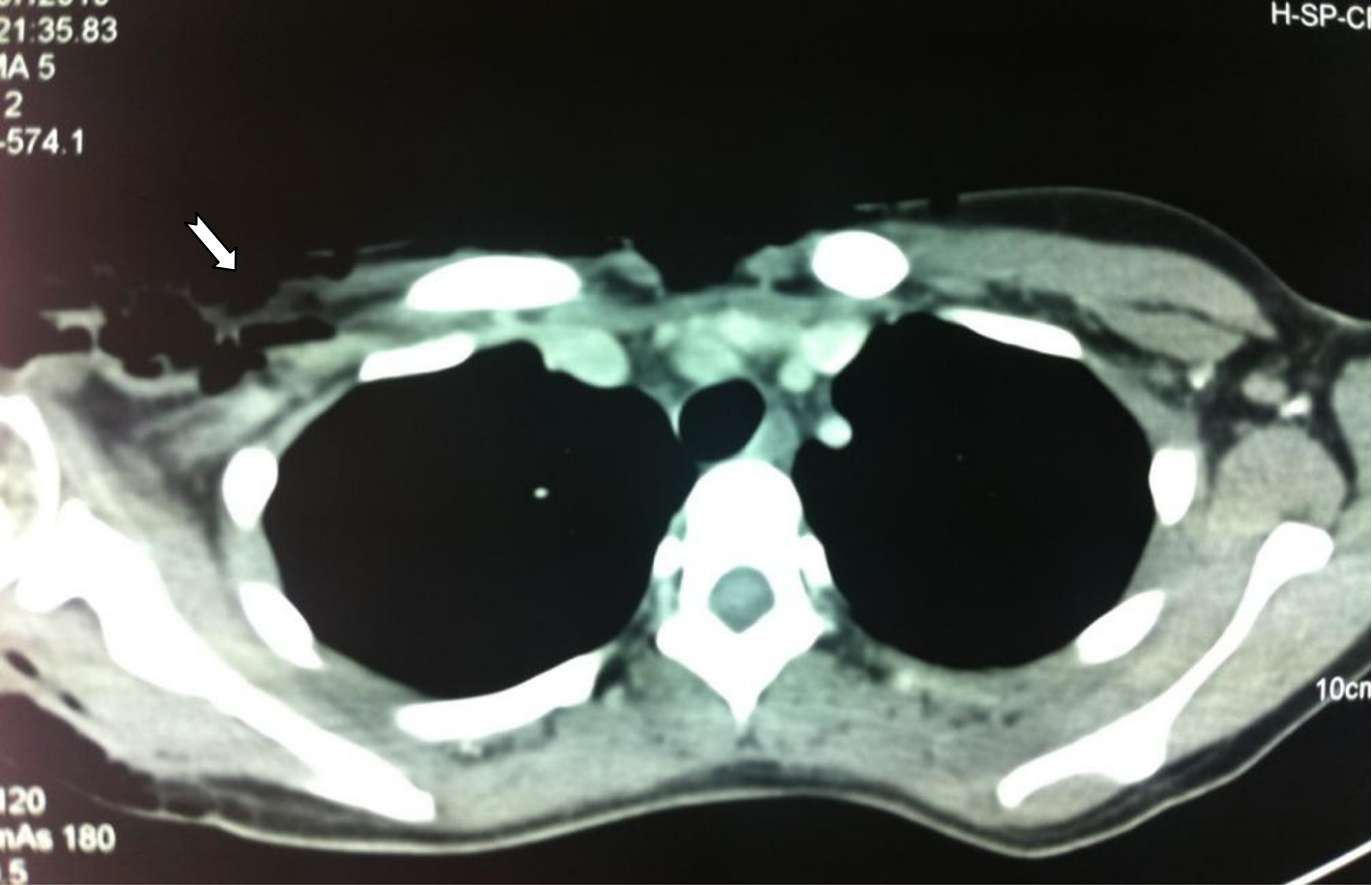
Thoracic computed tomography image showing a diffuse subcutaneous emphysema (arrow showing emphysema)
